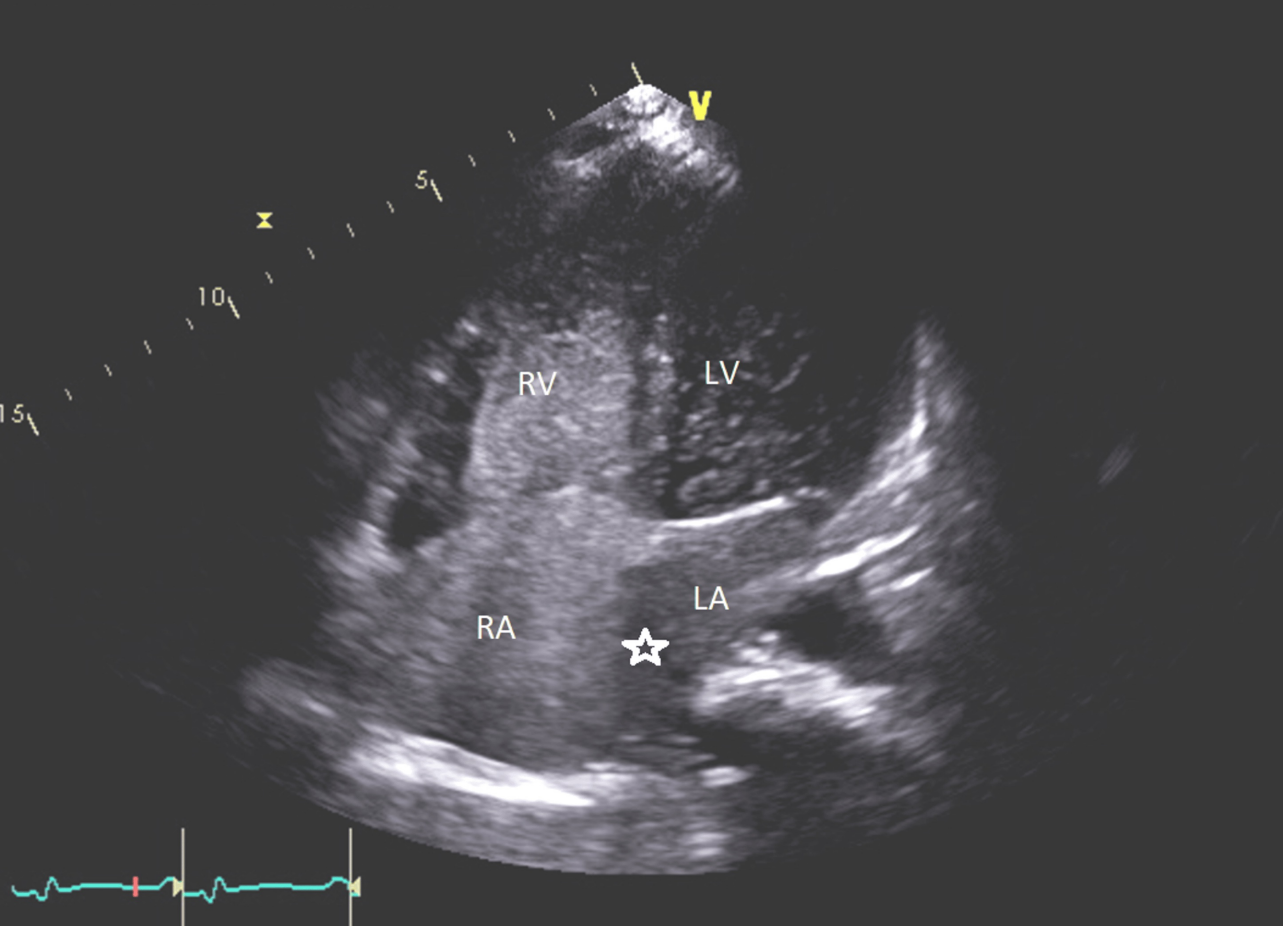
The apical four-chamber view on echocardiogram. Notice how the bubbles deviate from right atrium to left atrium indicating a positive bubble study. This demonstrates an interatrial septal defect. RA: Right atrium, RV: Right ventricle, LA: Left atrium, LV: Left ventricle, Star: Interatrial septal defect
